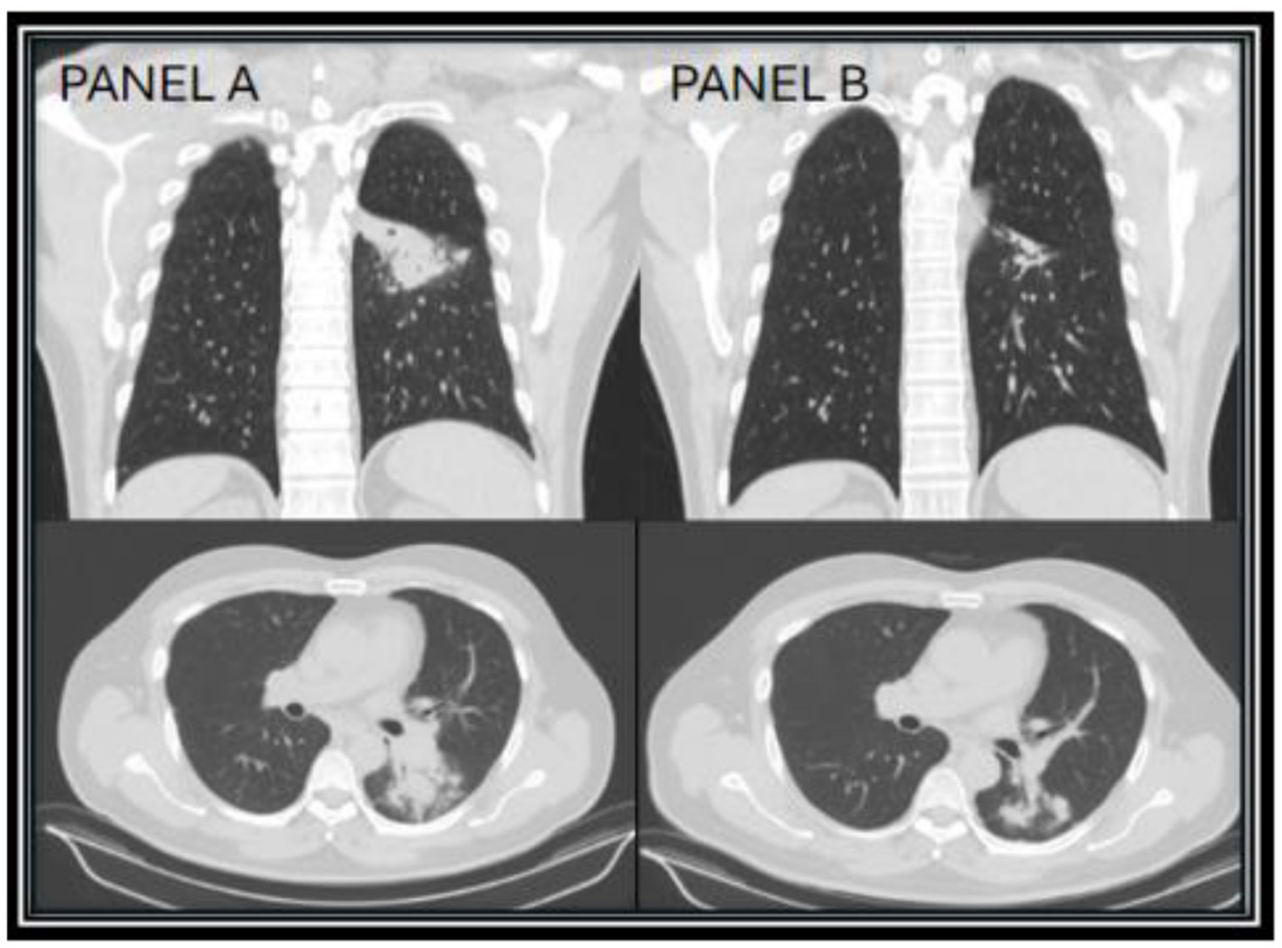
CT chest images taken 1 month after diagnosis (year zero) of Coccidioides infection showing the superior left lower lobe infiltrate (A), and repeat CT chest taken 17 months on itraconazole showing improvement of the superior left lower lobe infiltrate (B).
